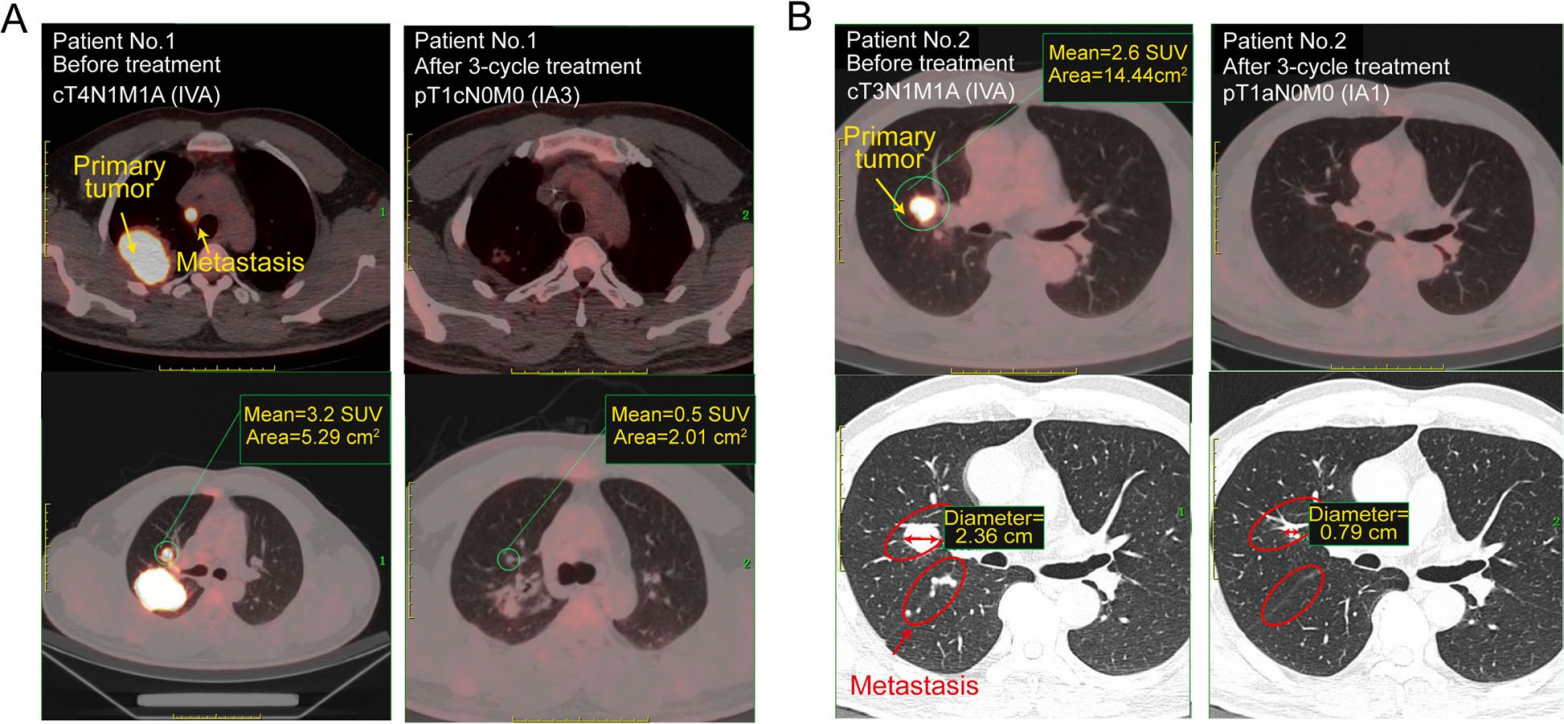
Positron emission tomography/Computed tomography (PET/CT) of representative NSCLC patients with metastasis before and after P-A combination therapy.
